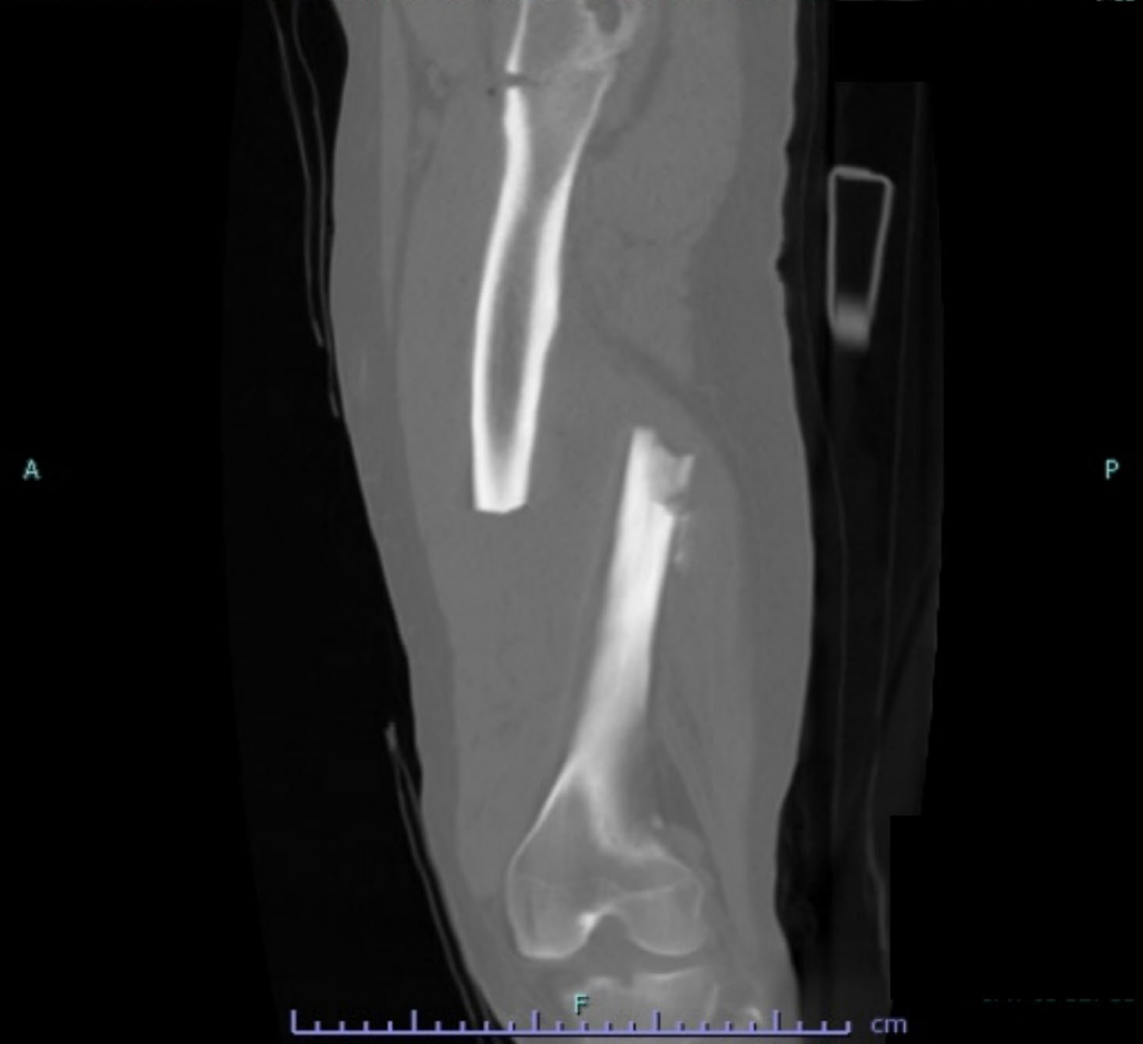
Initial left femur CT scan upon admission.
